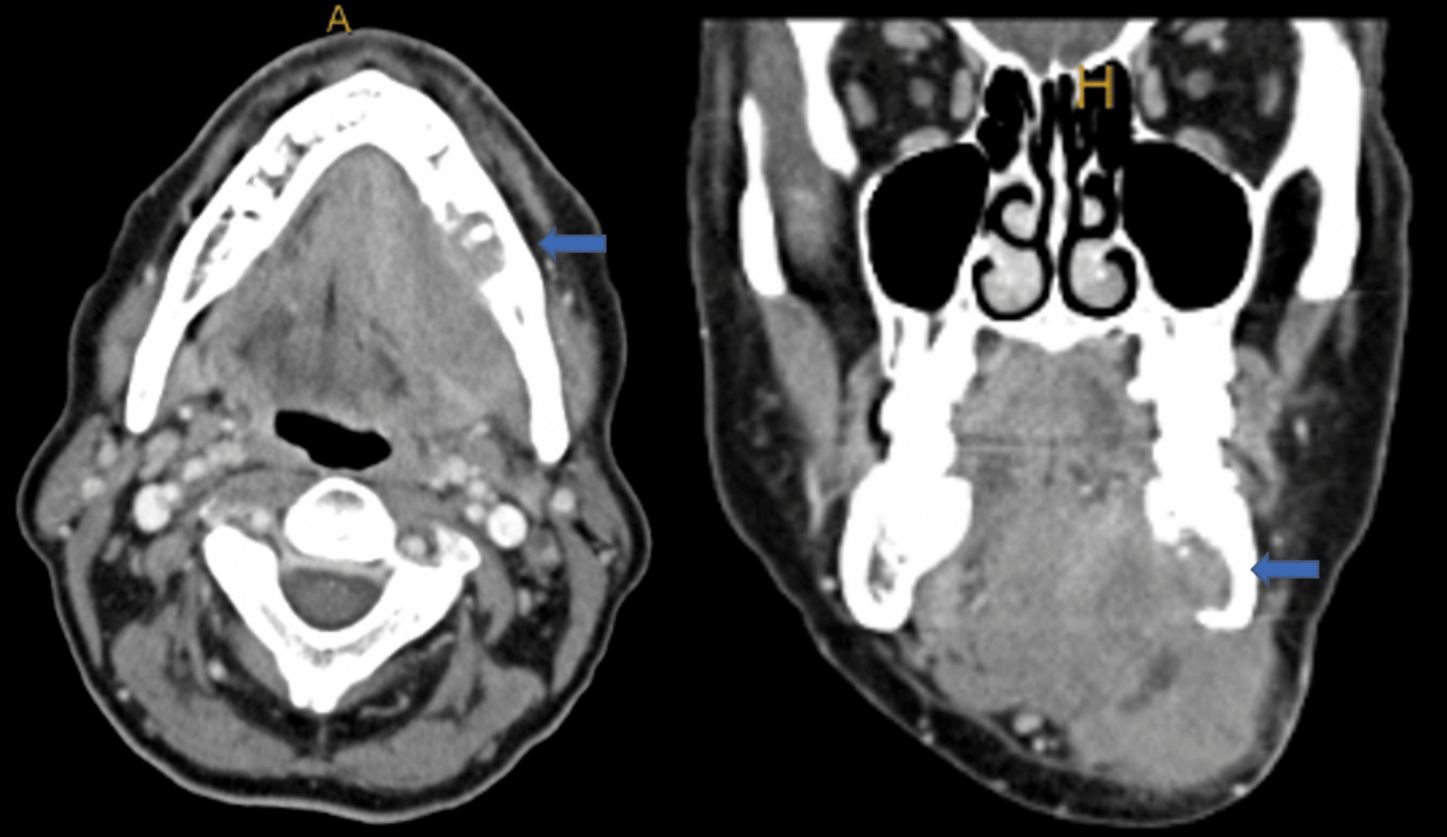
Pre-operative CT Pretreatment CT neck pre-op shows soft tissue mass breaking through the lingual cortex of the mandible. The blue arrow points to a mass involving the left mandibular cortex.
